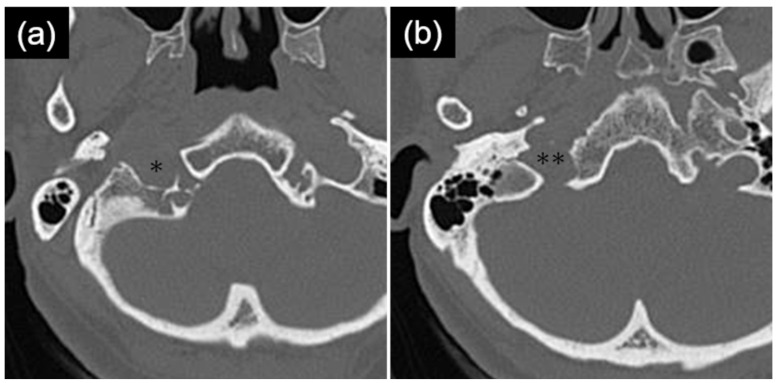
Thin-slice computed tomography with bone window settings. (a) Enlargement of the hypoglossal canal (*) is observed, most prominently along its lateral (extracranial) portion. (b) The enlarged hypoglossal canal is continuous with the expanded jugular foramen ().
